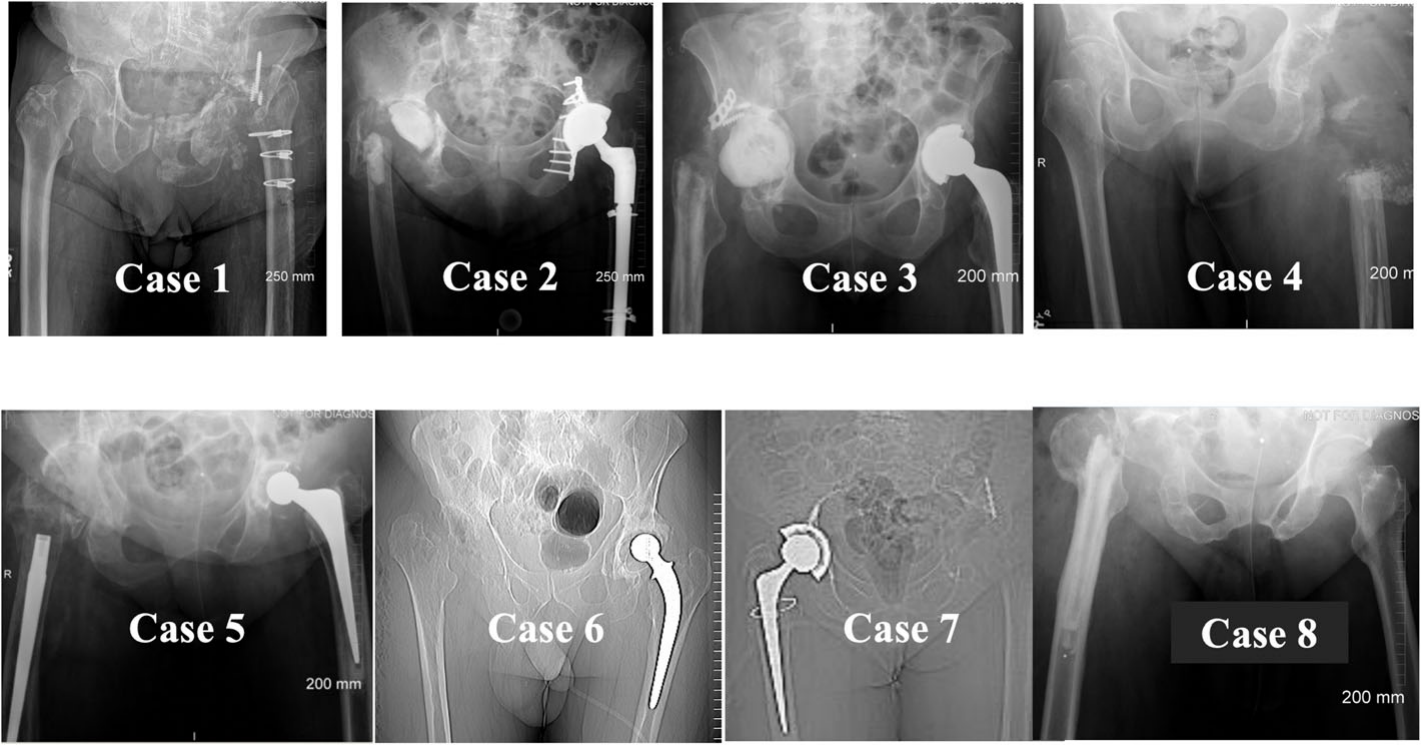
Series of radiographs taken after the removal of the implants showing the remaining bone stock. In Cases 2 and 3, the acetabular spacer can be seen
